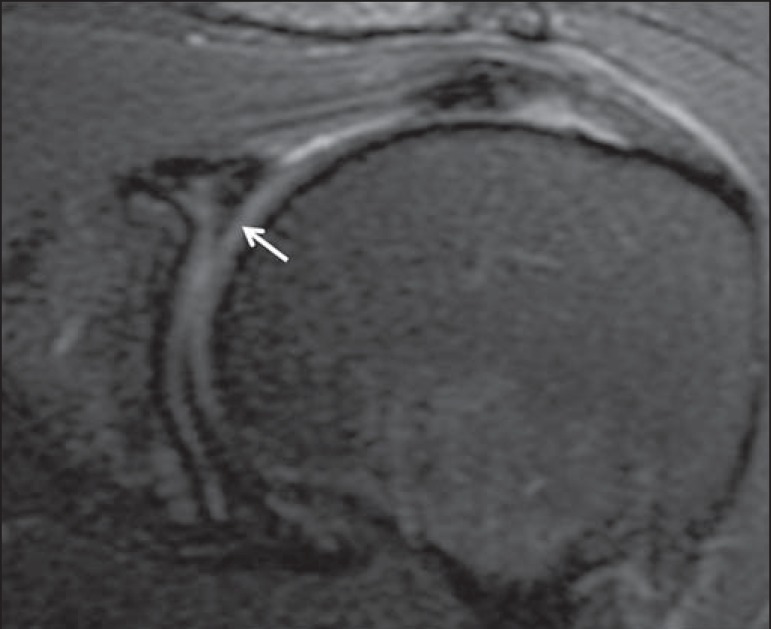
54-year-old female. Coronal T2-weighted MRI scan with fat saturation. The
